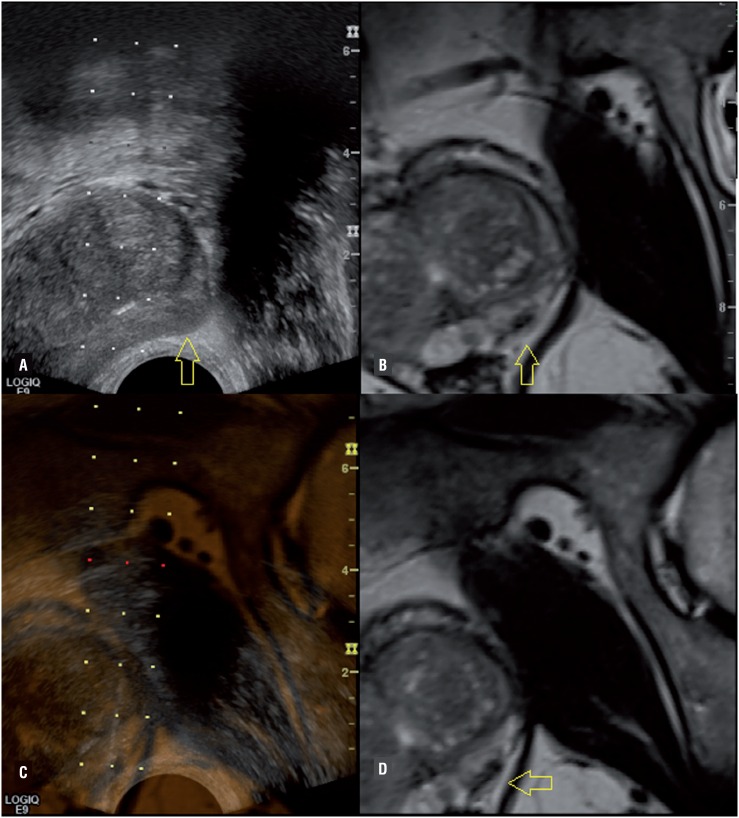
A suspicious lesion on mpMRI (images B and D) submitted to a US-MRI fusion biopsy. The lesion was not seen on US (arrow in A) and the biopsy was performed based on mpMRI (arrow in C). The biopsy result was Gleason 3+4 in all tree fragments of this area.
